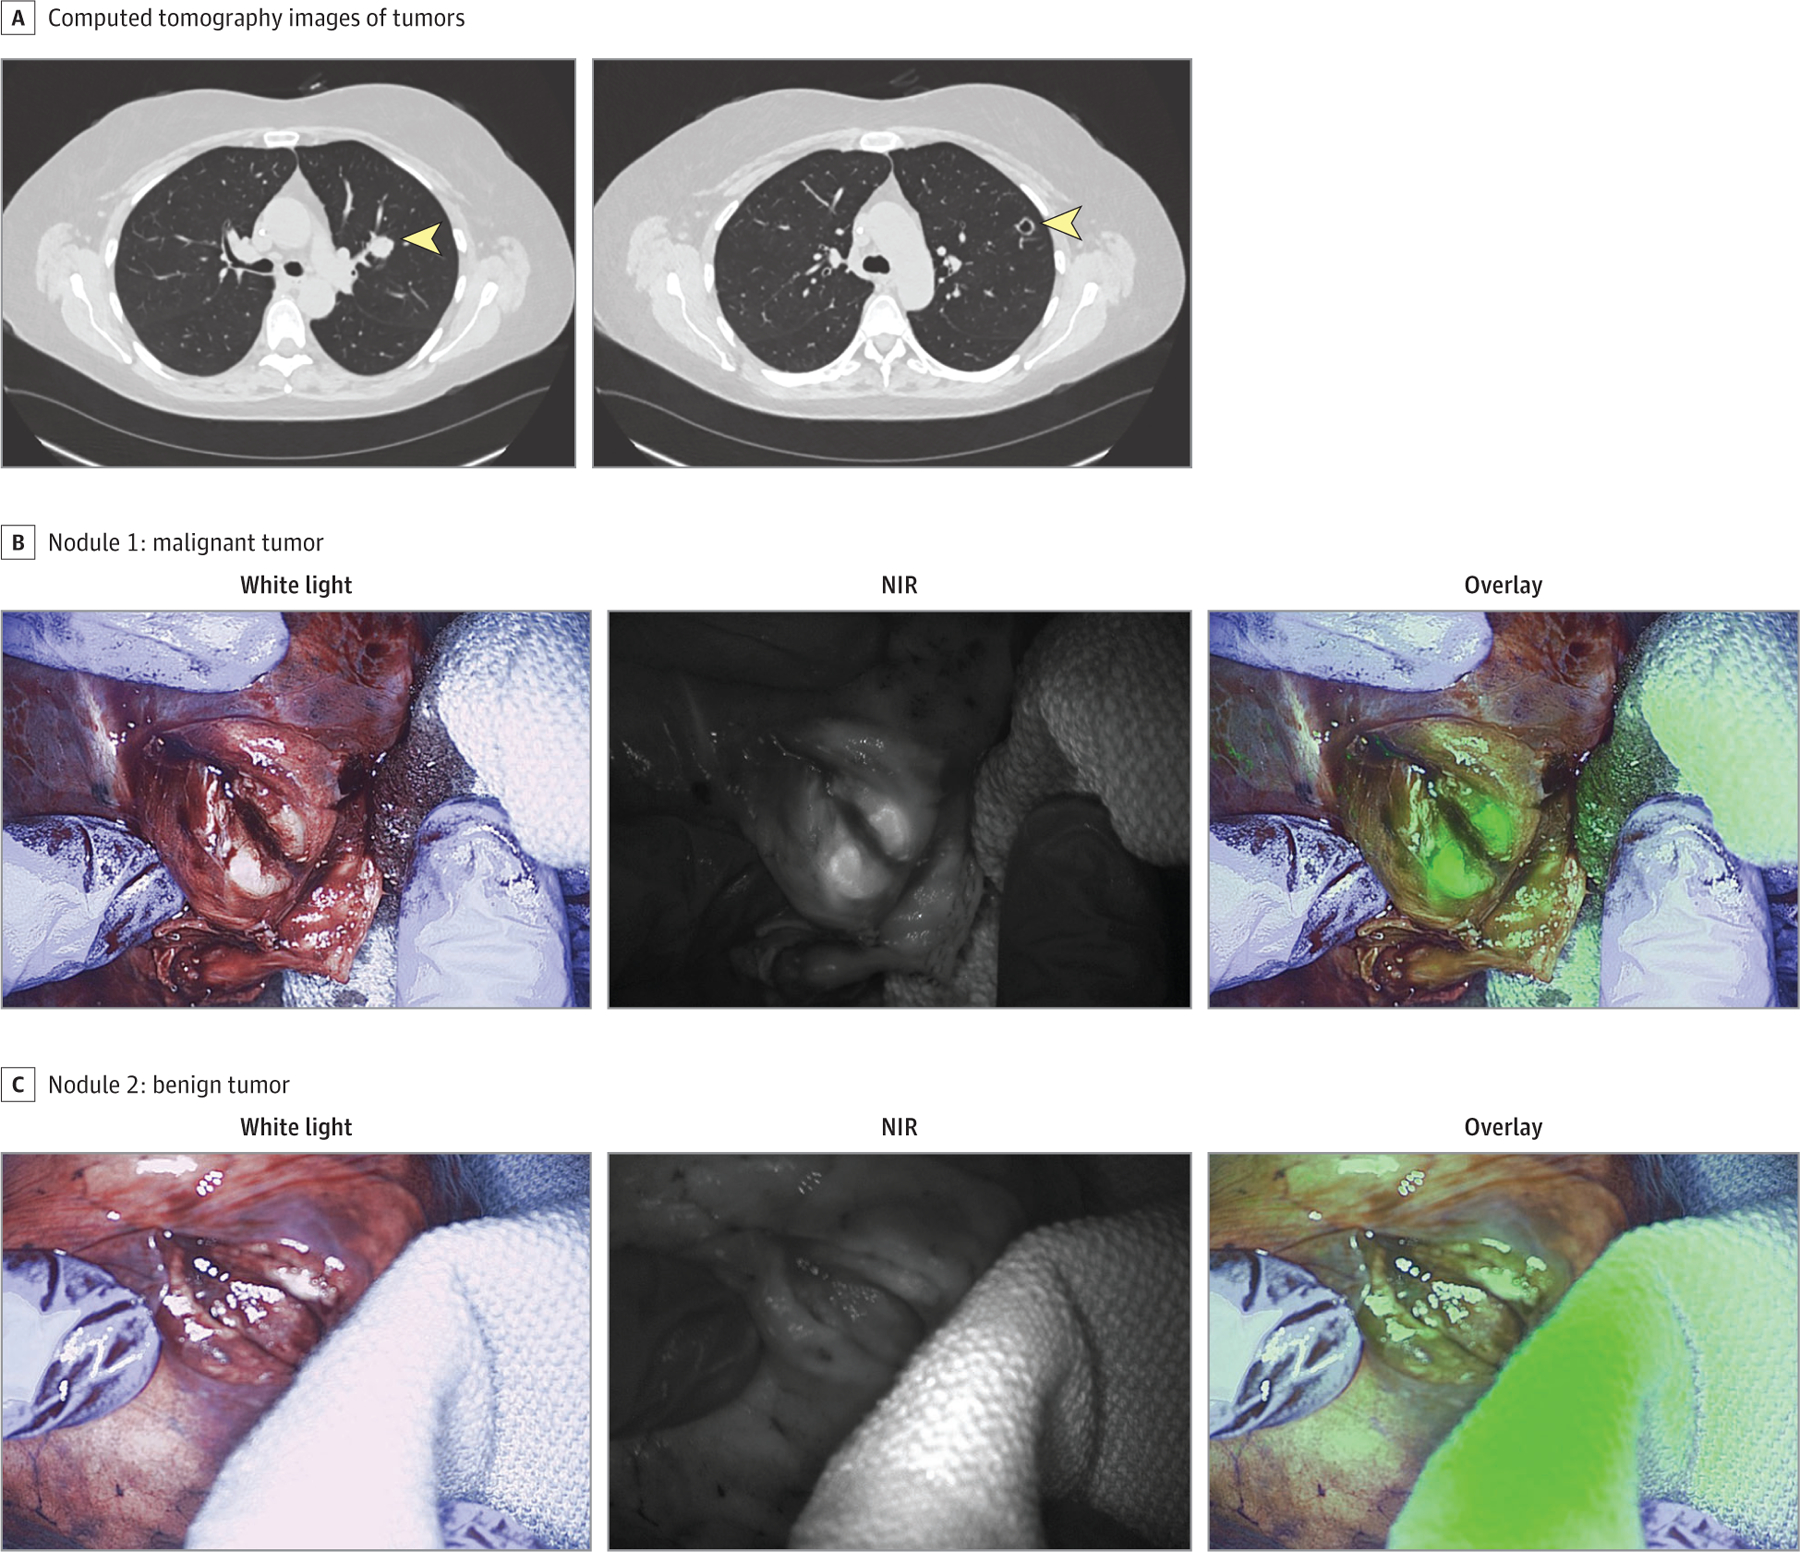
SGM-101–Guided Lung Nodule Resection in a Control Patient A, Computed tomography images of the tumor (yellow arrowheads indicate tumor). B, Nodule 1. C, Nodule 2. NIR indicates near infrared.
